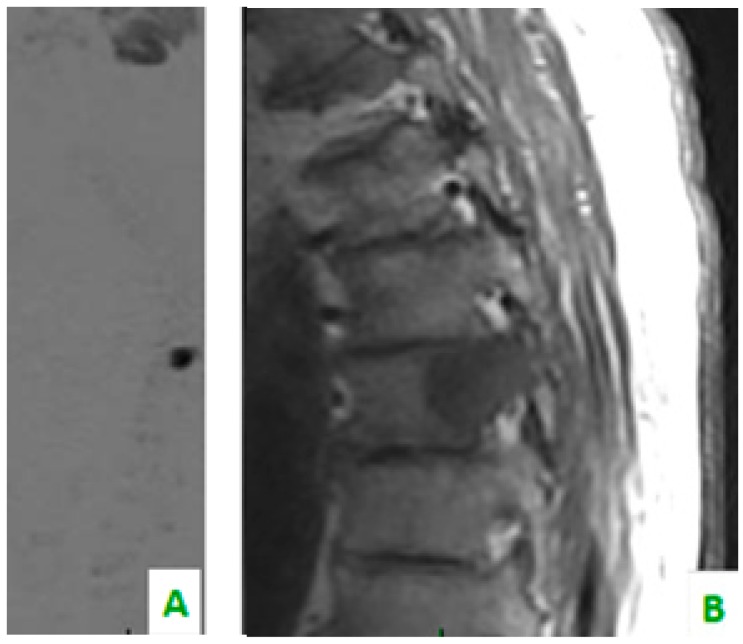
(A) Diffusion-weighted imaging (DWI) sequence in sagittal plane shows focal alteration of diffusivity. (B) The sagittal plane of TSE T1 shows, in the same level, a hypointense region of the dorsal soma related to the secondary localization of prostate cancer.
